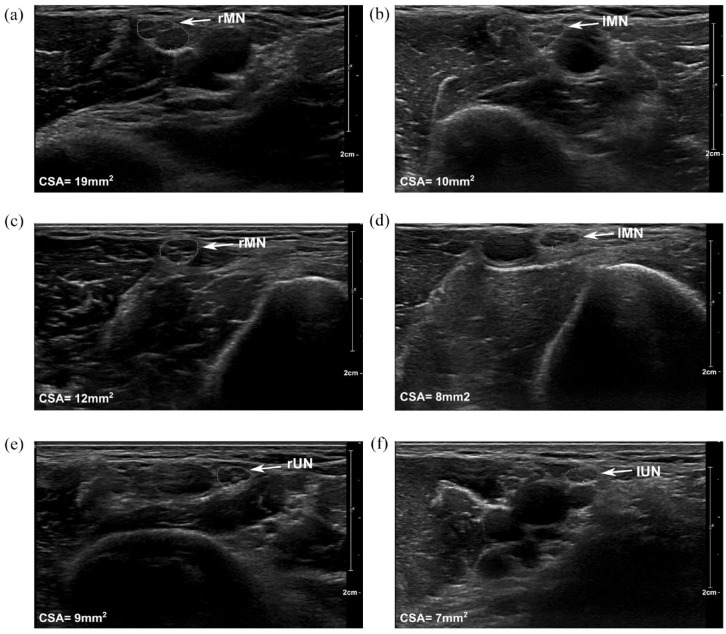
Ultrasonography shows enlargement of peripheral nerves. Ultrasonography of peripheral nerves in the right (a, c, e) and left (b, d, f) arm. Enlargement of right median nerve (rMN) in the proximal (a) and middle (c) upper arm compared to the left median nerve (lMN) (b, d). Enlargement of fascicles of the right (e) ulnar nerve (rUN) in the proximal upper arm compared to the left ulnar nerve (lUN) (f). Ultrasound depth depicted as a scale on the right, cross-sectional area (CSA) of the respective nerve on the bottom left. Circled area depicts the measured nerve.
